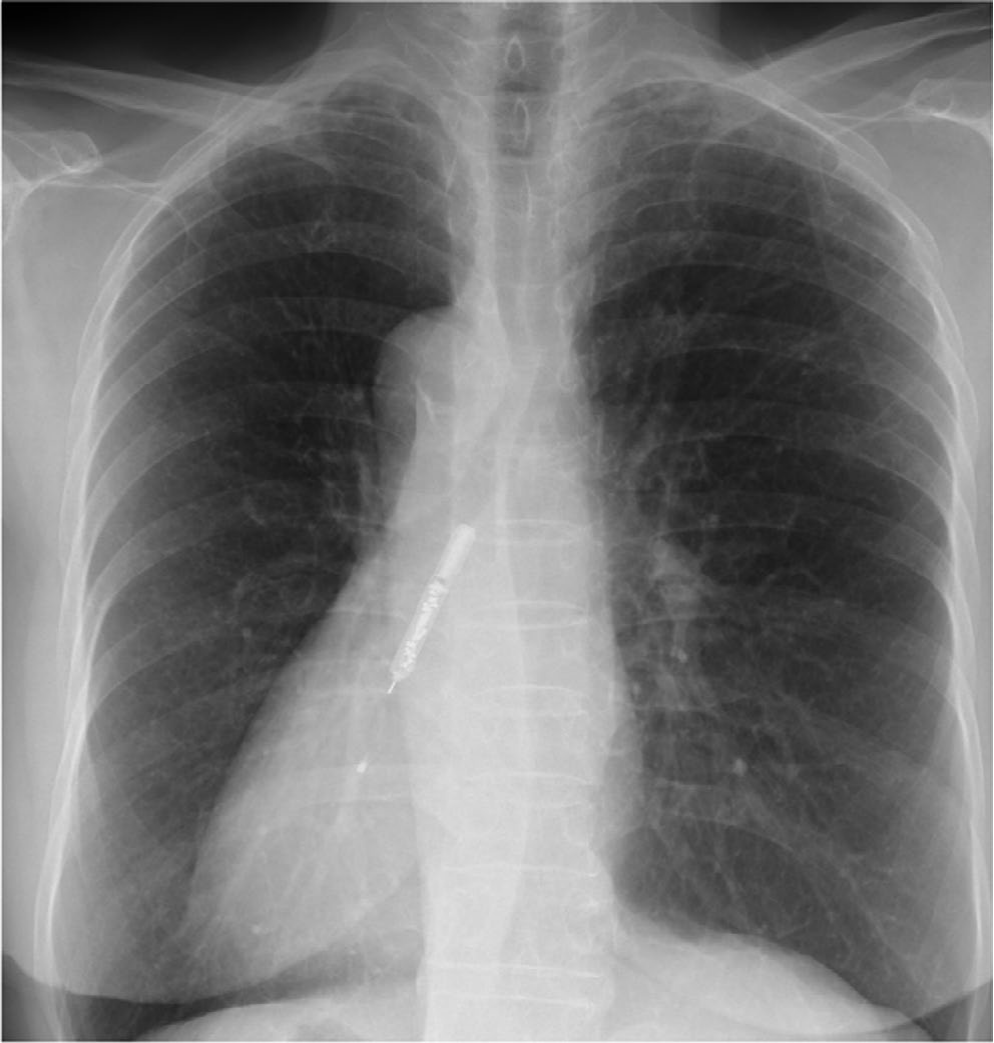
Chest radiograph shows cardiac apex points to the right, indicating situs inversus totalis. Insertable cardiac monitor can be seen in the right margin of the 3rd to 6th intercostal sternum along the long axis shadow of the heart
